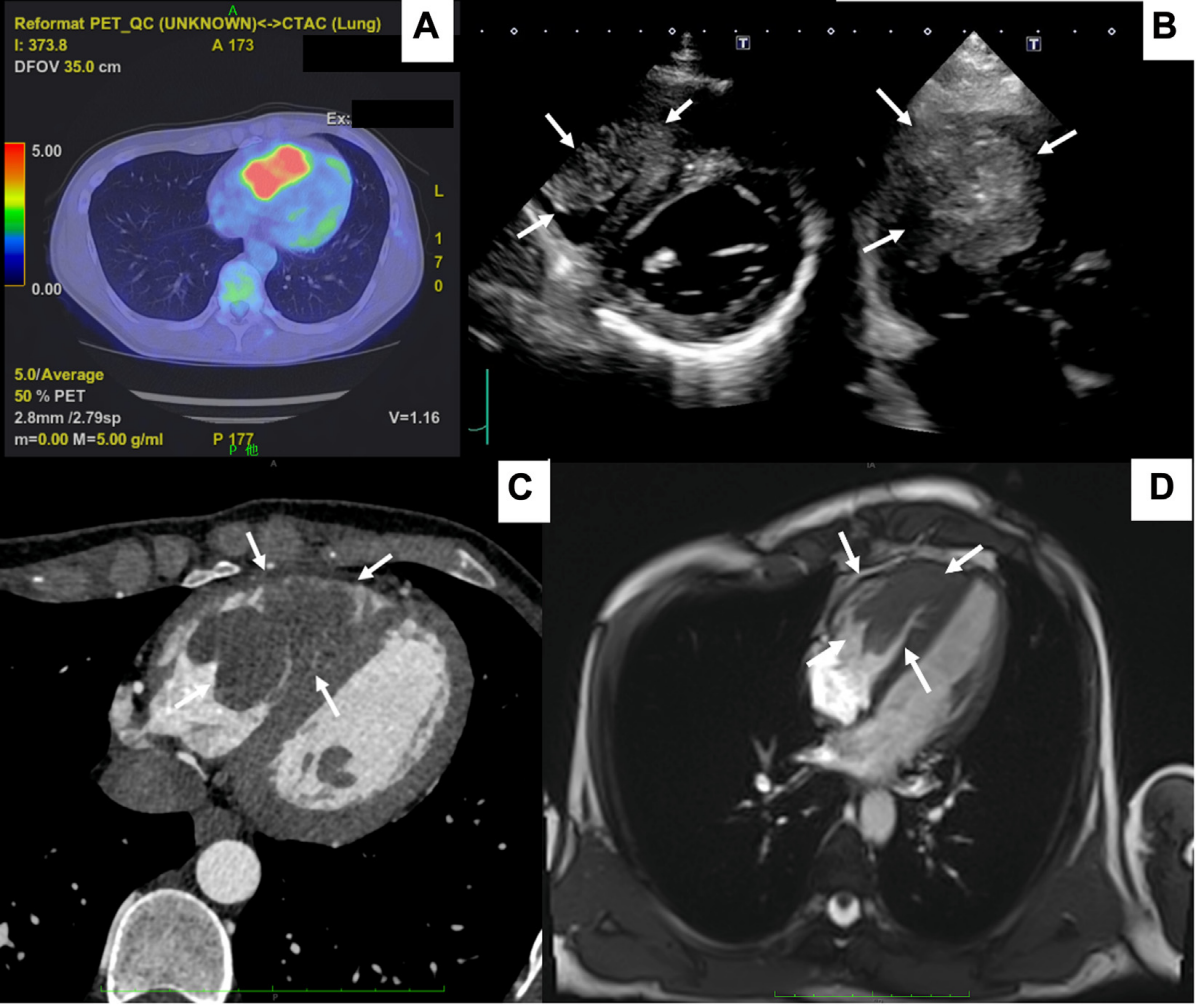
Multi-Modality Imaging of the Right Ventricle (A) Positron emission tomography–computed tomography showing fluorodeoxyglucose accumulation in the right ventricle. (B) Preoperative echocardiography. (C) Contrast-enhanced computed tomography revealing a low-density mass in the right ventricle. (D) Short T1 inversion recovery mode of cardiac magnetic resonance displaying a low-density mass in the right ventricle. White arrows indicate the metastatic tumor.
